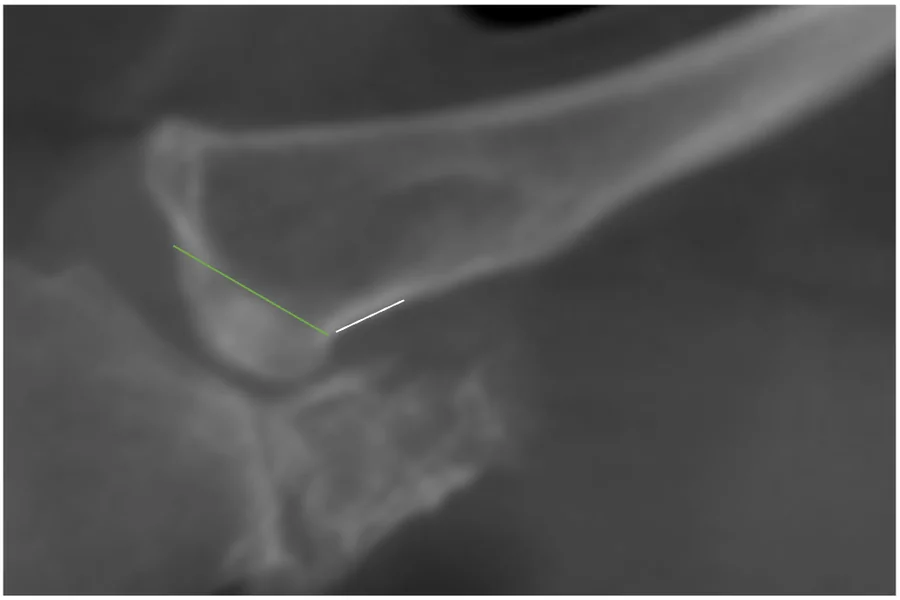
Preoperative dual-energy computed tomography of a left SCJ. The resection includes the inferior two-thirds of the medial clavicle (green line). During this, care is taken to preserve the costoclavicular ligament, which inserts approximately 10 mm lateral to the inferior sternoclavicular articular margin on the inferior aspect of the clavicle. SCJ, sternoclavicular joint.
